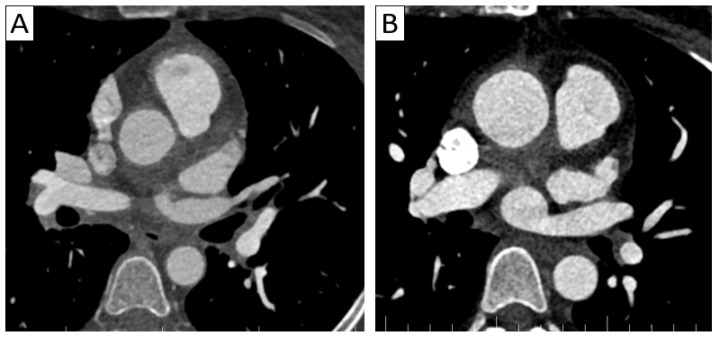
(A) A 37-year-old patient with Takayasu arteritis presenting with unstable angina. Axial thoracic CTA demonstrated aortic wall thickening and periaortic infiltration, as well as parietal inflammation of the supra-aortic trunks and coronary arteries, with myocardial infarction in the left anterior descending coronary artery territory. (B) A 35-year-old patient with Takayasu arteritis presenting with severe aortic insufficiency—aortitis was suspicioned intraoperatively during valvular replacement. Axial thoracic CTA demonstrated parietal thickening of the ascending aorta and periaortic infiltration.
